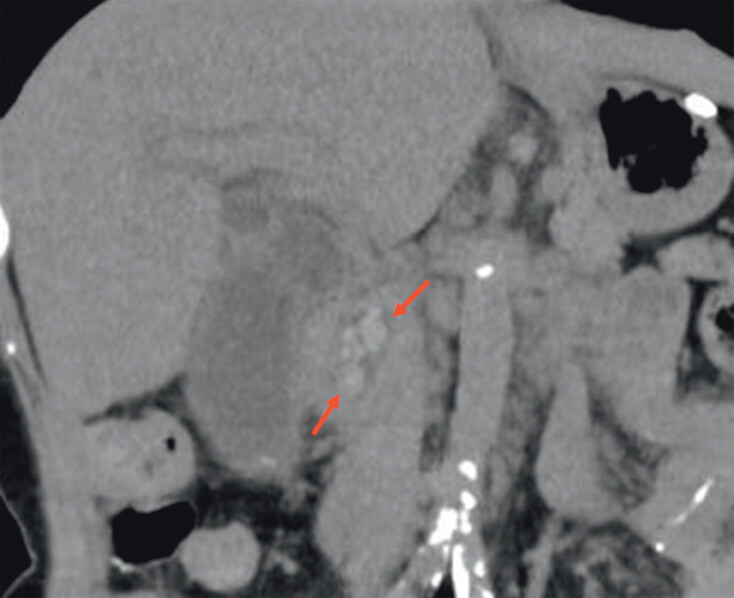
Computed tomography revealing multiple stones in the common
